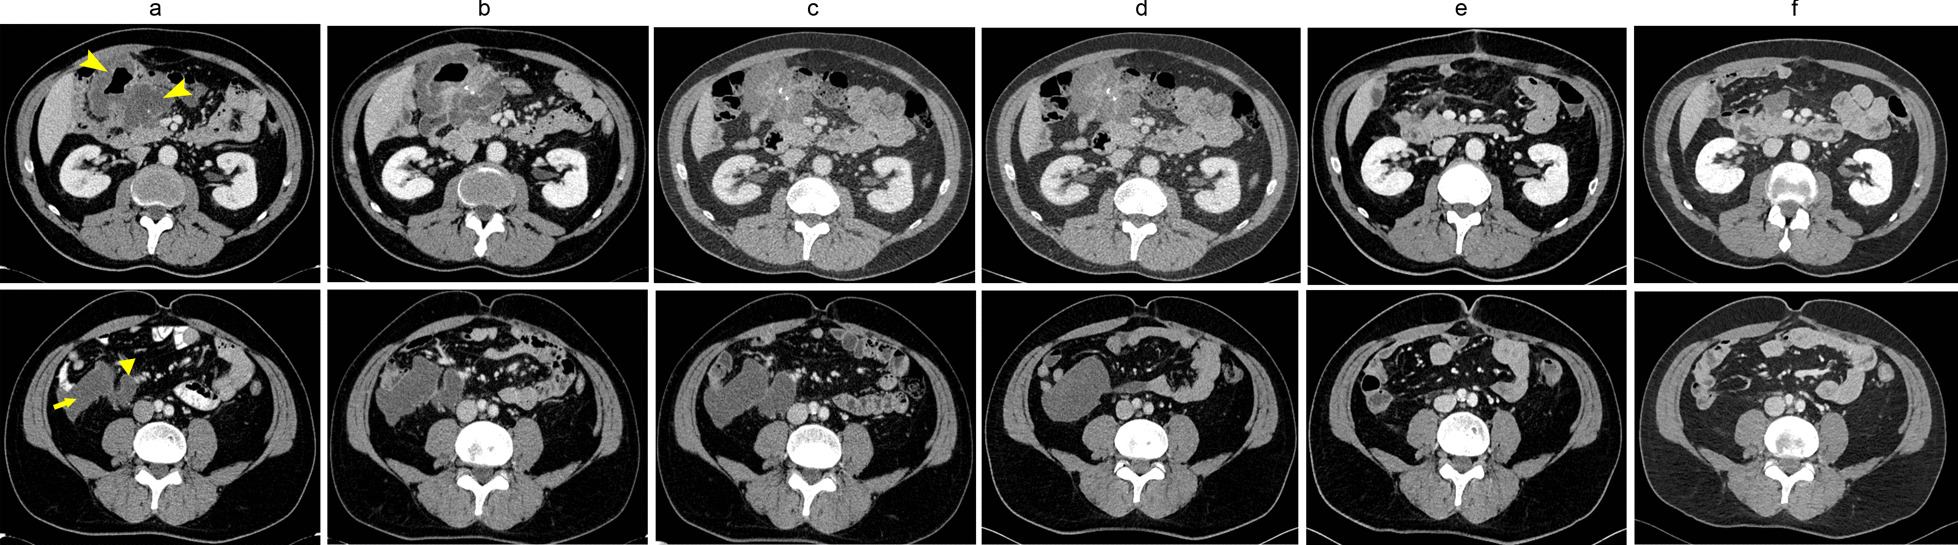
Title: Imaging (CT-scan) evolution of peritoneal metastases. Seriated CT-scan images: appearance of perianastomotic (top row) and right parieto-colic gutter region (lower row). a Recurrence diagnosis (May 2018), b after first-line (XELOX-Bevacizumab, July 2018), c after 2 months of Nivolumab (October 2018), d after 18 months of Nivolumab (February 2020), e three months after cytoreductive surgery (June 2020), f nine months after surgery (December 2020). Arrowheads: perianastomotic lesion, arrow: parieto-colic lesion, triangle: superior vessels nodal lesion
